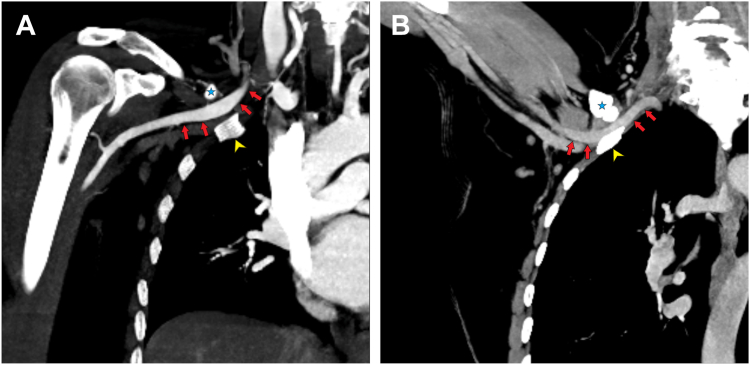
Coronal MIP CTA images showing normal arterial patency the arm adducted (A) and showing arterial compression the arm abducted/elevated (B) of the right subclavian artery (*redarrows*) between the hypertrophic clavicular pseudarthrosis (*blue star*) and first rib (*yellow arrowhead*). *MIP*, Maximum Intensity Projection; *CTA*, computed tomography angiography.
